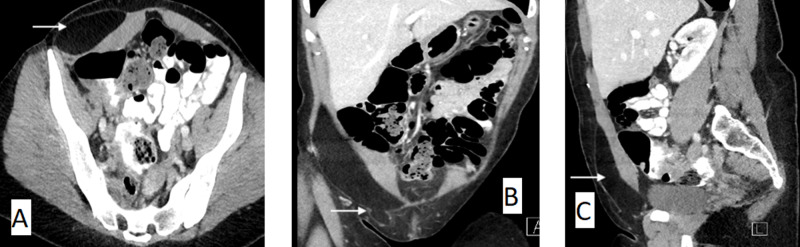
Axial (A), coronal (B), and sagittal (C) view of a CT scan of the abdomen and pelvis with arrows demonstrating a large right anterior abdominal wall lipoma arising between the external and internal oblique muscles extending into the inguinal canal.
